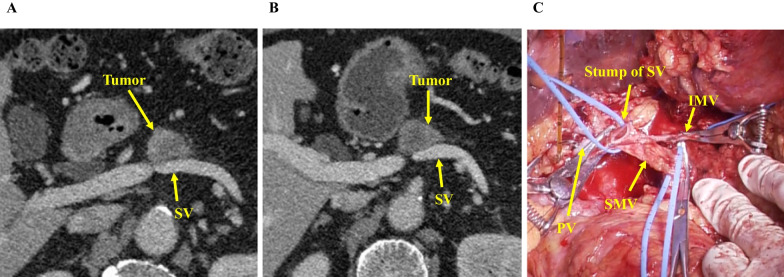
CT before the surgery and operative findings. A CT before neoadjuvant chemotherapy showing the tumor near the SV. B CT after chemotherapy showing that the tumor is almost the same size as that before chemotherapy and located close to the SV. C We resected the SV at the confluence of the PV. *CT* Computed tomography, *SV* Splenic vein, *PV* Portal vein, *SMV* Superior mesenteric vein, *IMV* Inferior mesenteric vein
